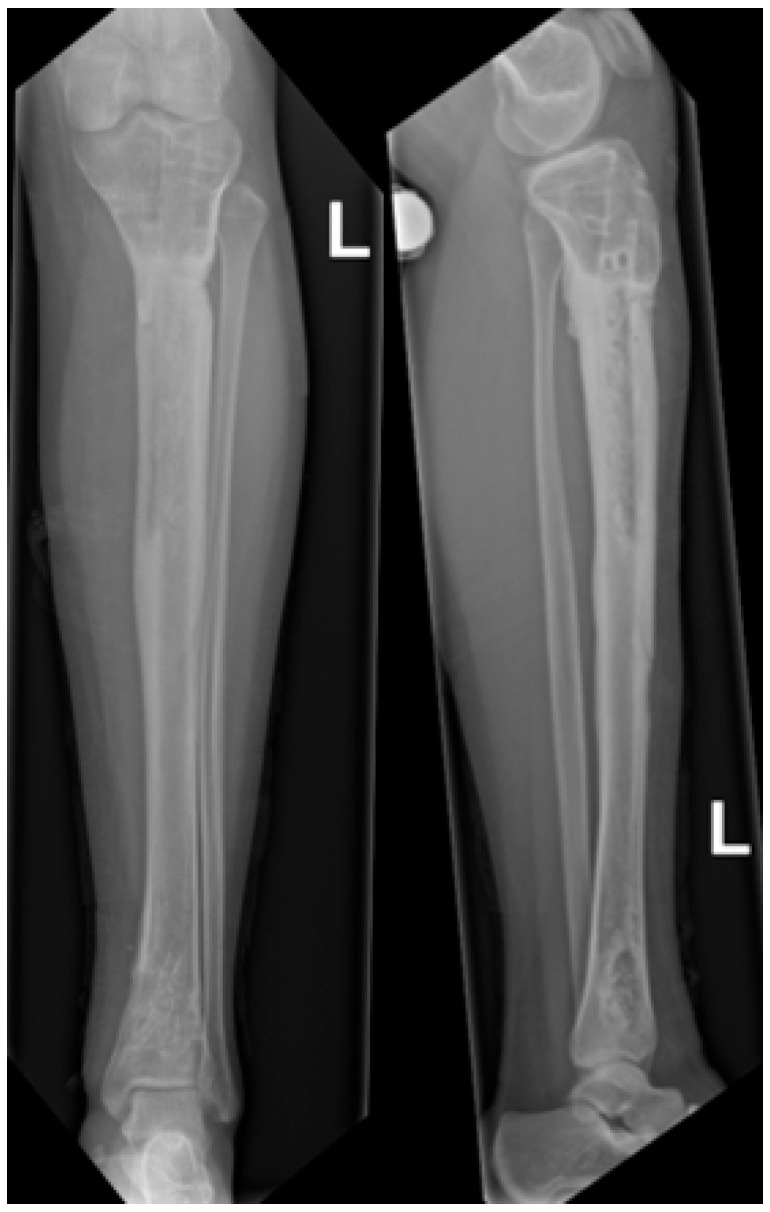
Radiographs following hardware removal, showing anteroposterior view (left) and lateral view (right) of the tibia. Slight procurvatum and varus alignment are noted.
